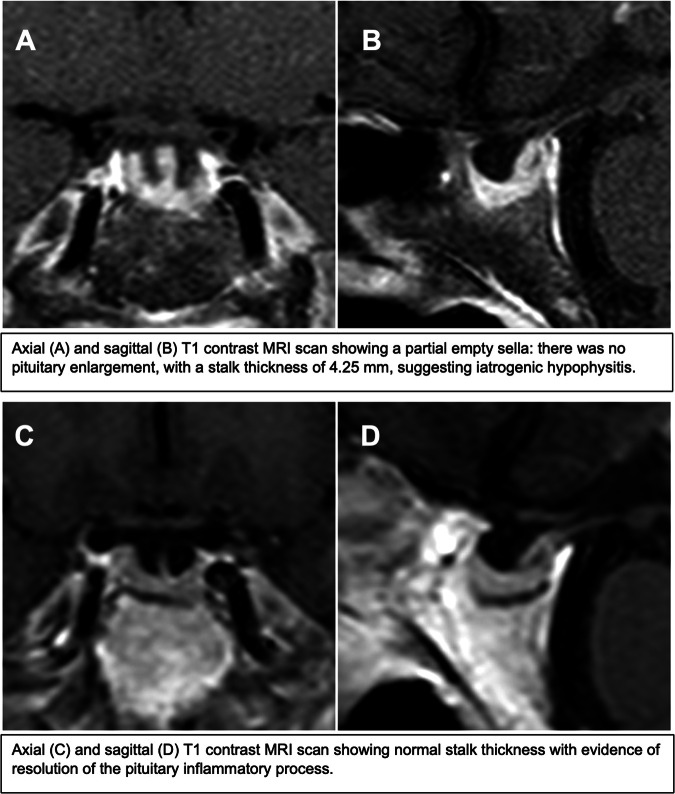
Pituitary MRI. August 2019 (adrenal crisis): panel (A, B); January 2020: panel (C, D). MRI magnetic resonance imaging
